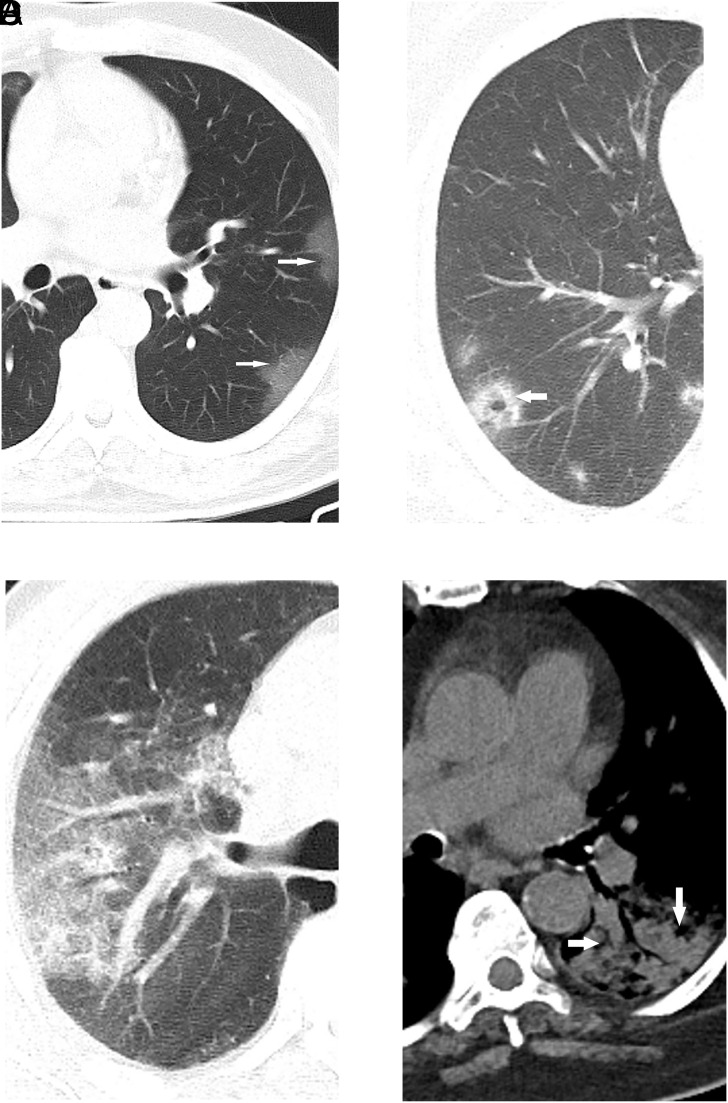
(A-D) Common CT involvement patterns in COVID-19 pneumonia. (A) Peripheral ground-glass opacity (arrows). (B) Reverse-halo sign is defined as a focal, rounded area of ground-glass attenuation surrounded by a ring of denser consolidation (arrow). (C) Crazy-paving refers to the appearance of ground-glass opacity with superimposed interlobular septal thickening and intralobular septal thickening. (D) Consolidation with air bronchograms (arrow). CT, computed tomography; COVID-19, coronavirus disease 2019.
